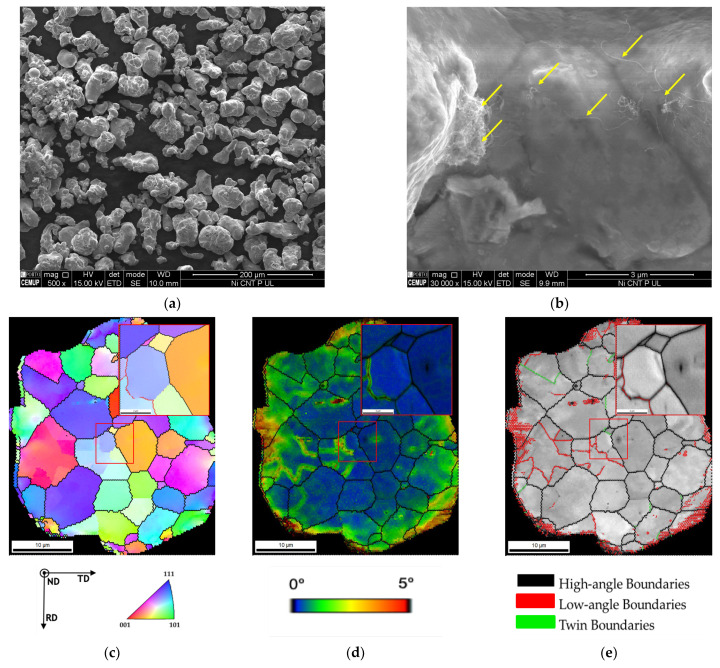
(a,b) SEM images of Ni-CNT particles after ultrasonication showing the presence of CNTs (yellow arrows) and (c) Inverse pole figure (IPF) map, (d) Kernel average misorientation (KAM), and (e) image quality (IQ) with high- and low-angle boundaries and twin boundaries delineated.
